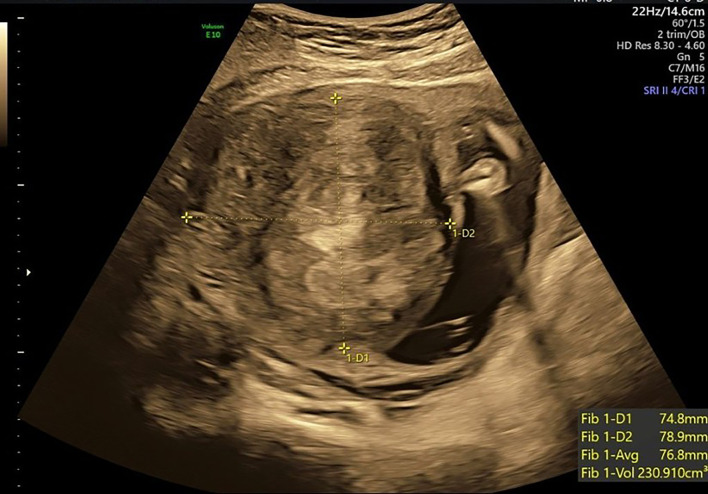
Prenatal ultrasound at 20 weeks’ gestation showing an anterior-wall intramural fibroid (yellow cross-marks) measuring approximately 7.5 × 7.9 cm. The lesion was in the lower anterior uterine segment.
